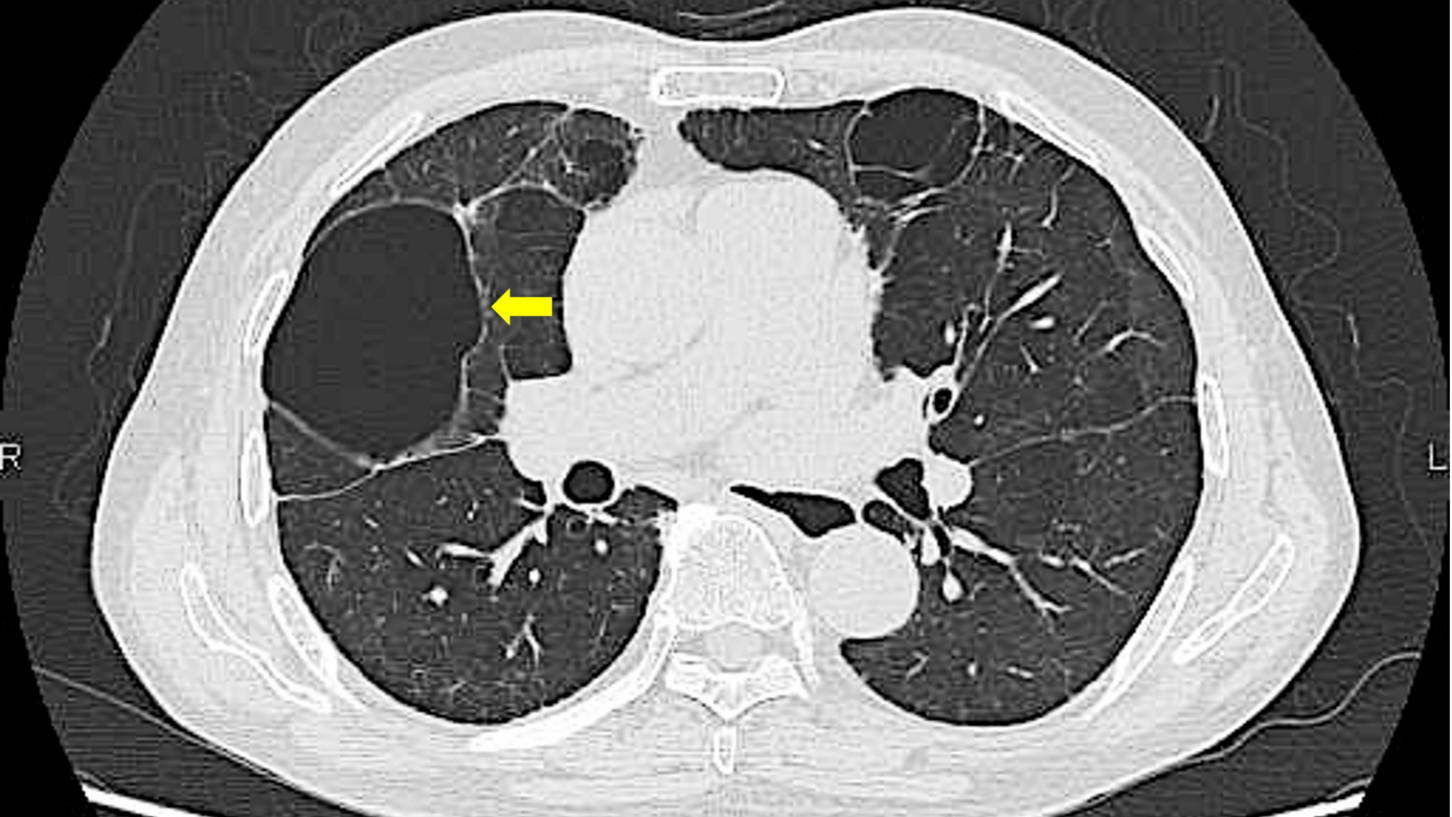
Chest CT scan six months after discharge showing the residual pneumatocele (arrow).
